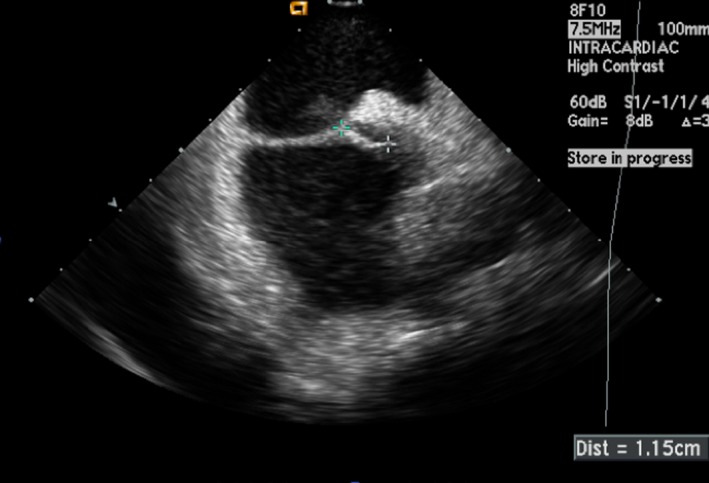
Intracardiac echocardiogram showing a patent foramen ovale (PFO) with a tunnel length of 1.15 cm.
